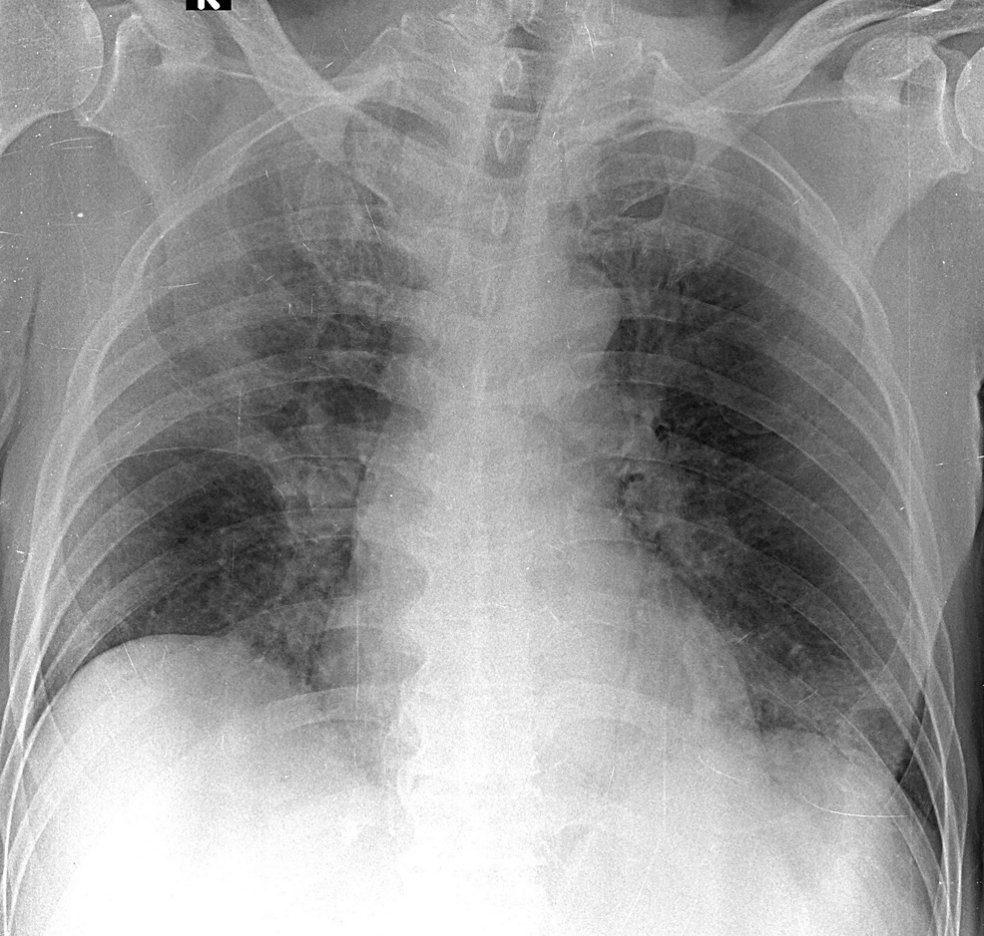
Atypical radiographic pattern in a moderate SARS-CoV-2 pneumonia case This CXR showed ground-glass opacity in the right peripheral lower zone and consolidations in the right upper lobe and left basal zone. The Brixia score was 12, the RALE score was 4, and the mCXR score was 8. This case was classified as moderate pneumonia based on clinical severity. The qCSI was 2.
